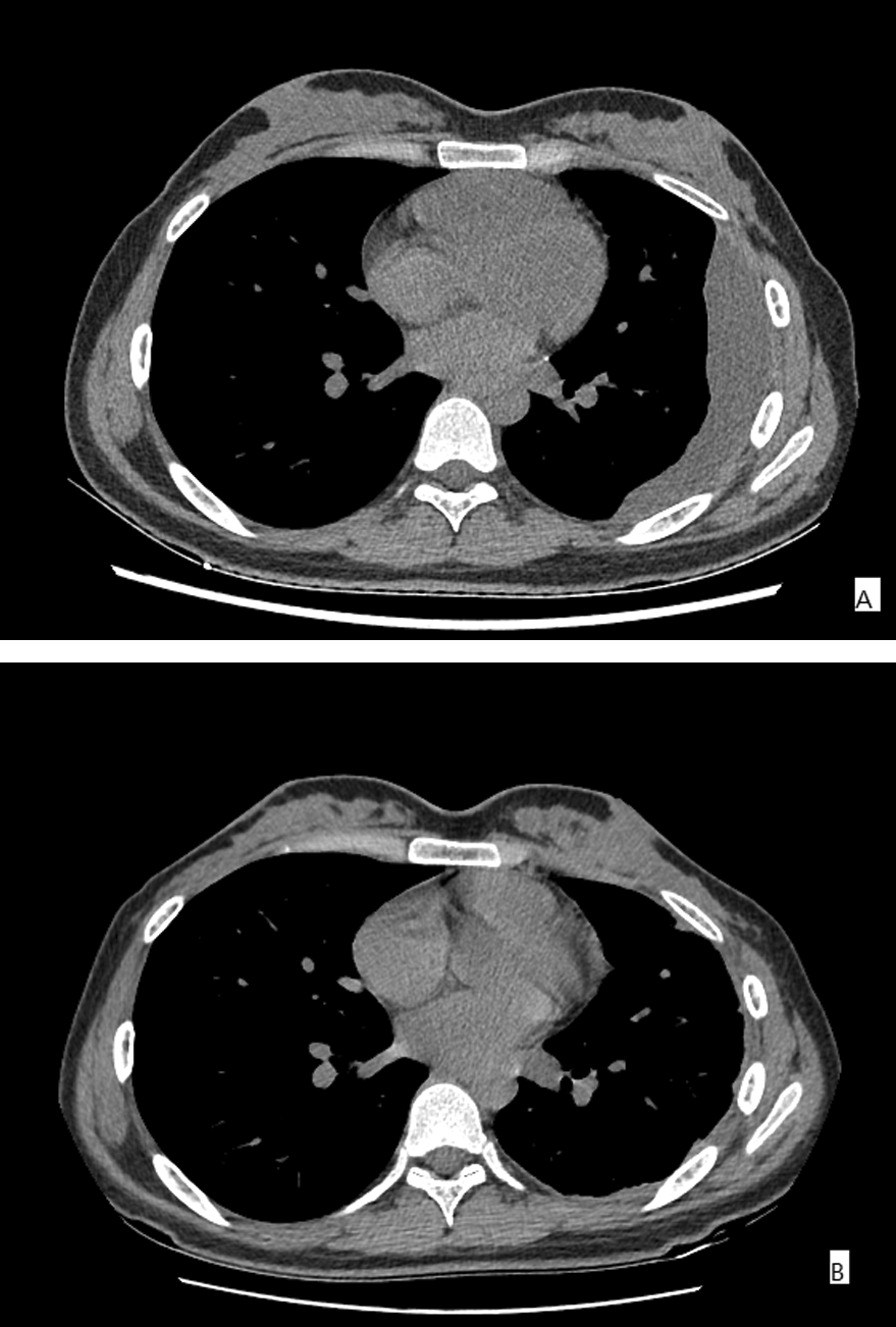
CT scan for the patient with tuberculous empyema. A CT for the patient with tuberculous empyema before operation. B CT for the patient after surgery for 6 months. *CT* computer tomography
